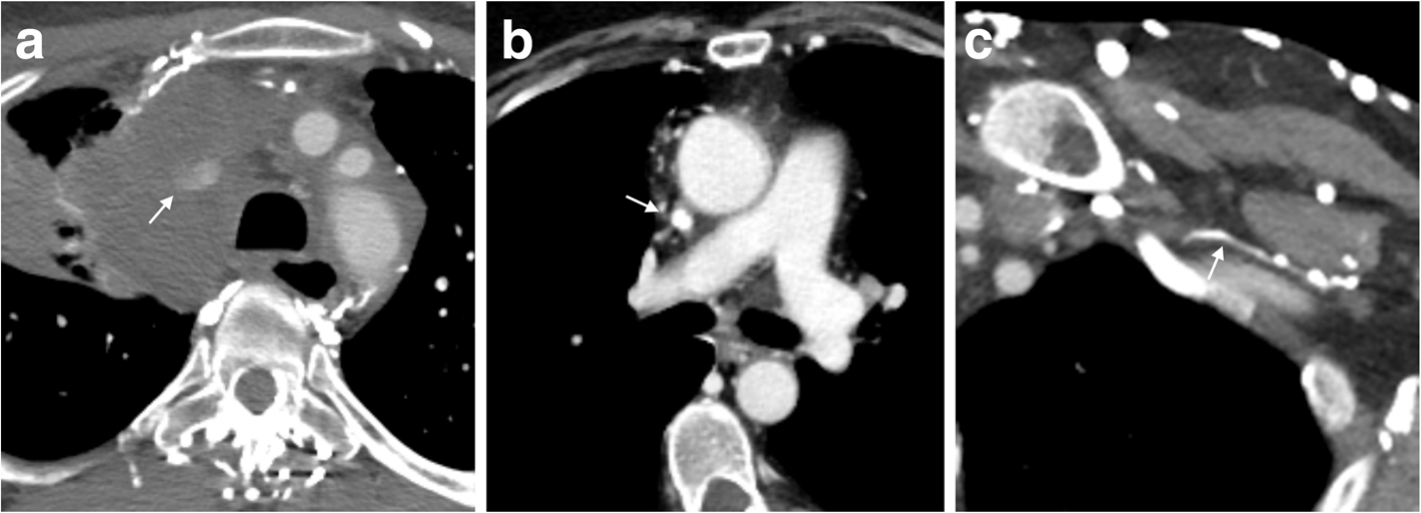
Causes of obstruction. a Axial CT image showing complete obstruction of the SVC secondary to extrinsic mass compression (arrow). b Axial CT image demonstrating SVC narrowing/occlusion (arrow) secondary to chronic indwelling catheter. c Axial CT image demonstrating intraluminal obstruction of the left axillary vein from a chronic thrombus (arrow)
